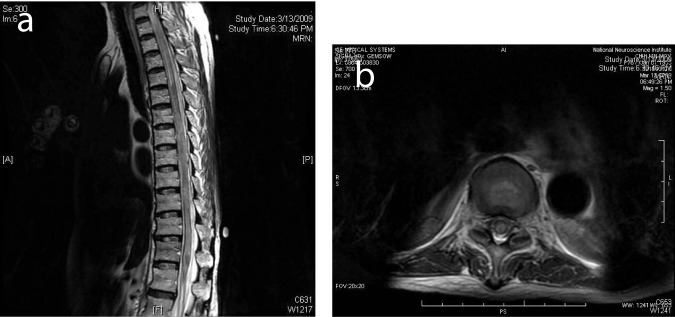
(a) MRI of the spine 1 week after presentation showed diffuse high T2 signal from the spinal cord from T6 to the conus compatible with cord edema. (b) Selected corresponding axial MR image showed edema across the whole cross-section of the spinal cord.
